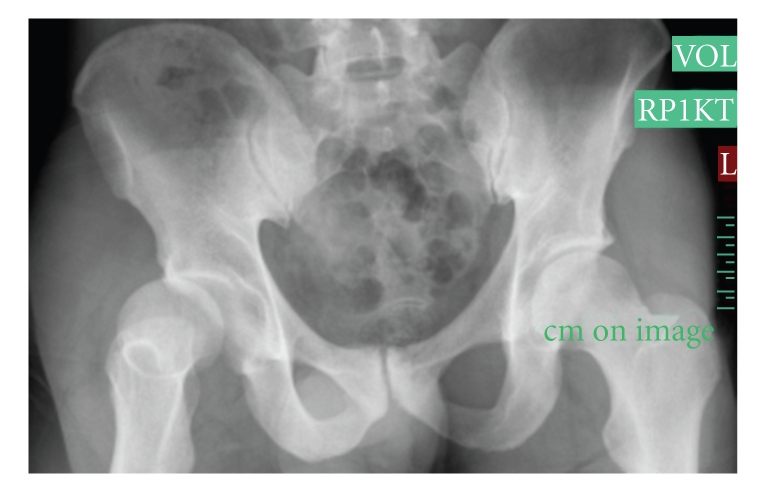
Plain radiograph of the pelvis shows a dislocated right hip.
